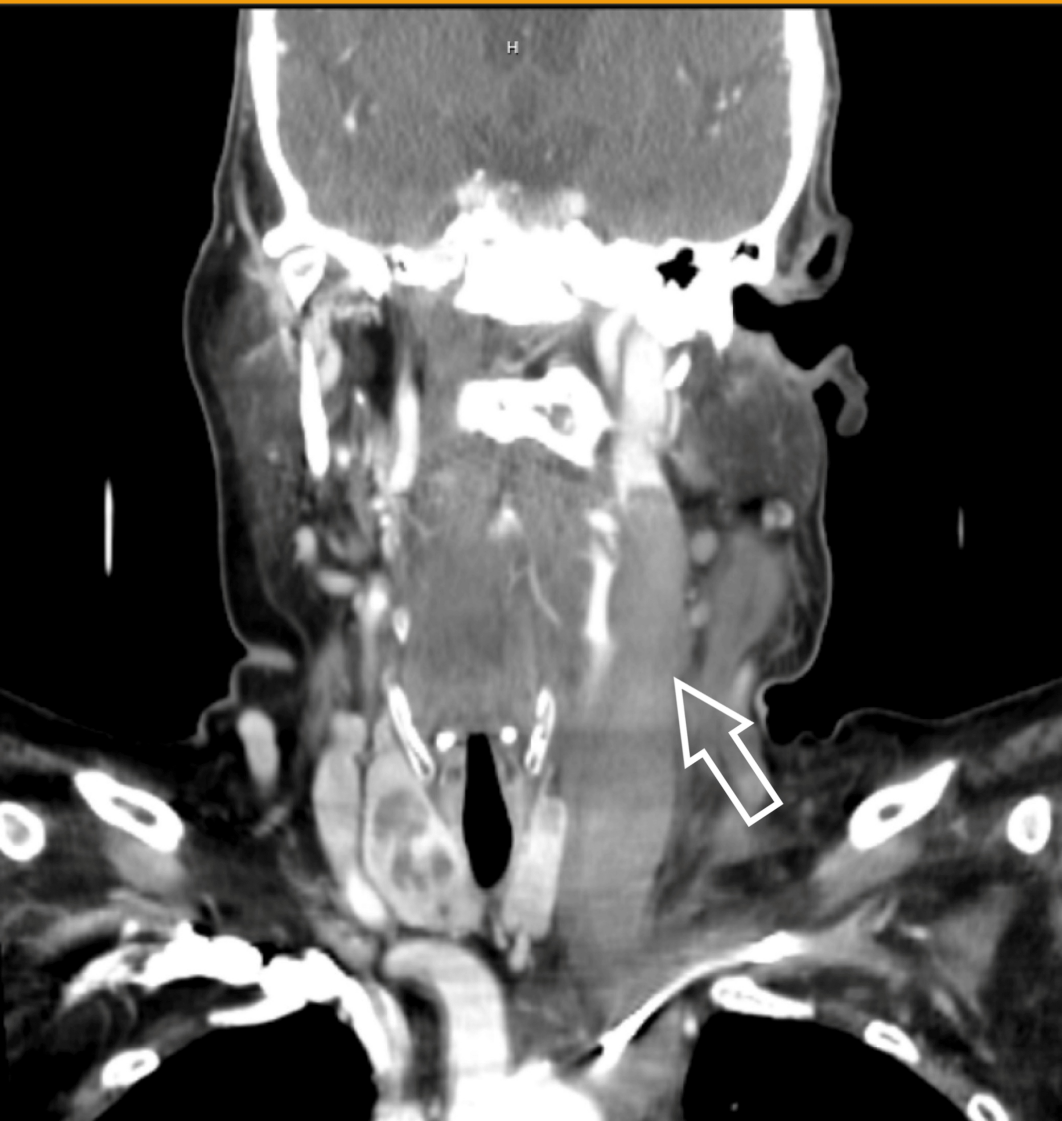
Thrombus of the internal jugular vein (white arrow) as seen on the coronal view of the computed tomography angiography (CTA) head and neck
